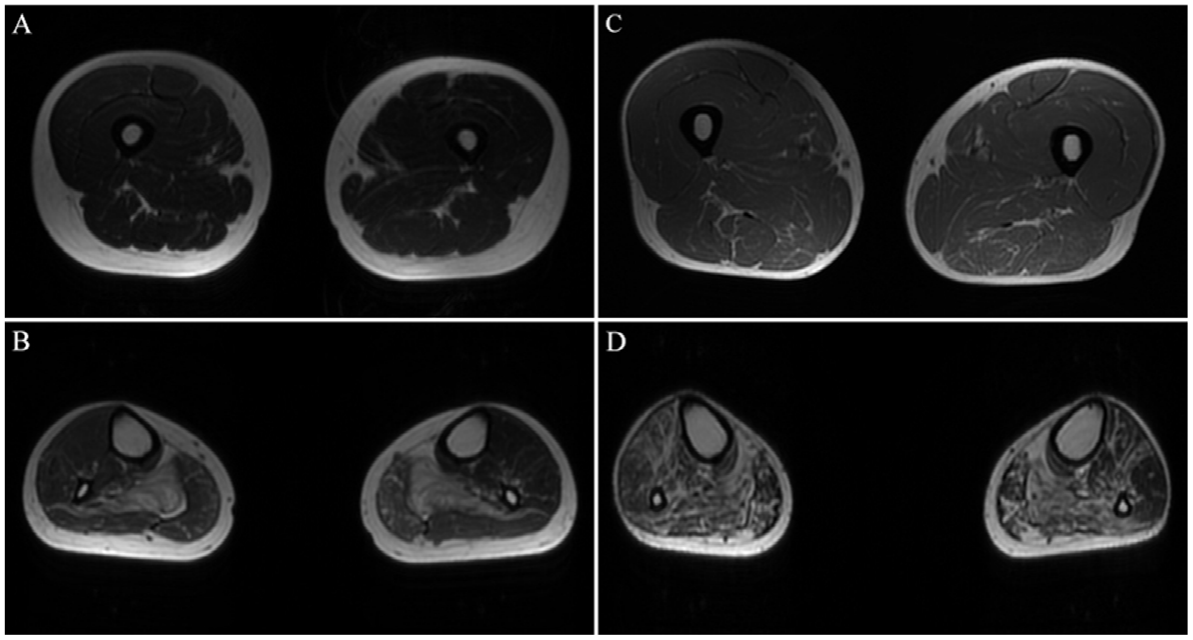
Axial T1-weighted MR images of patient 10 and patient 11. (A) and (B), Patient 10; (C) and (D), Patient 11. Both patients exhibited serious fatty replacement in the lower limb muscles, with particular involvement of the soleus. Only mild abnormalities were noticed in the quadriceps of patient 11.
